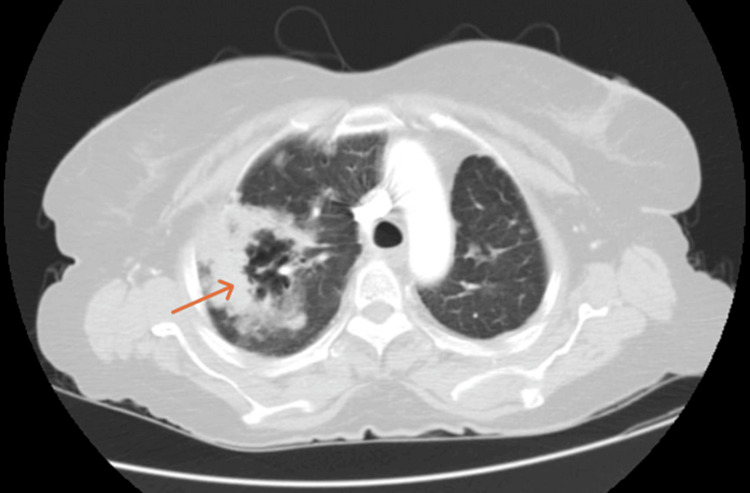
Contrast-enhanced computed tomography of the thorax showing cavitary lesion in the right upper lobe with perilesional consolidative changes (orange arrow)
